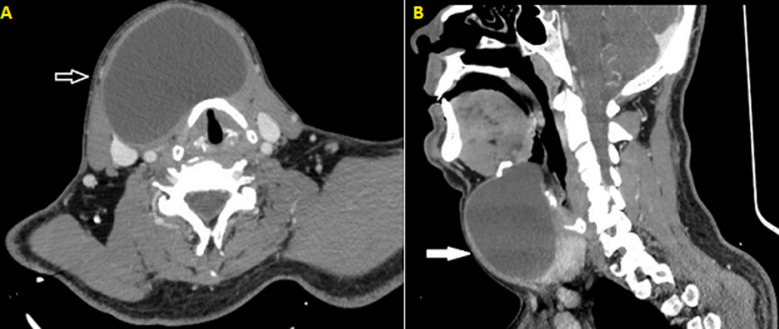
preoperative neck computed tomography of the reported case: A) axial scan; the arrow indicates the thyroglossal duct cyst measuring 8.7 cm axially and 6.6 cm anteroposteriorly; B) sagittal scan; the arrow indicates the cyst extending throughout the anterior neck space just below the hyoid bone
